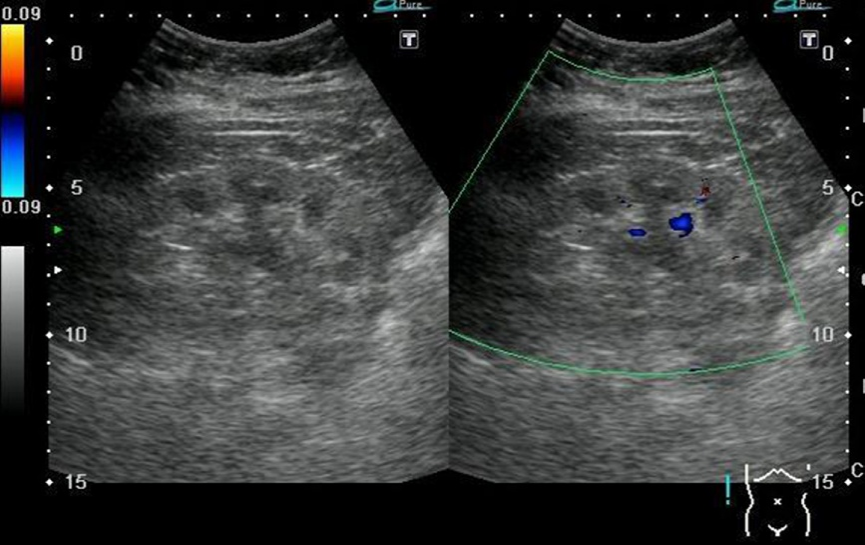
Post-operative ultrasonography. Ultrasonography of kidney showed mild hydronephrosis on right kidney.
